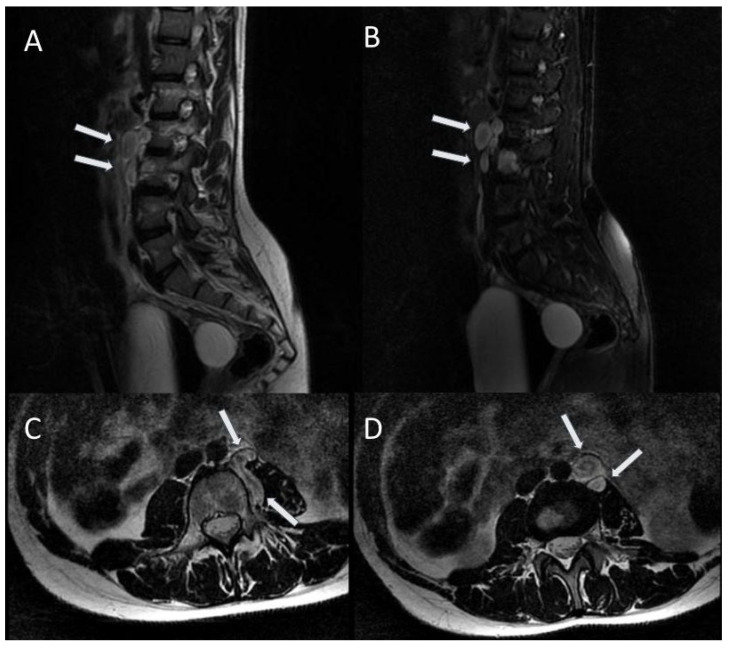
Left prevertebral and paravertebral neurofibromas (arrows) along the L2–L3 vertebrae. Sagittal T2-weighted FRFSE (A); sagittal T2-weighted FRFSE with fat suppression (B); axial T2-weighted FSE (C); axial T2-weighted FSE (D) [11].
